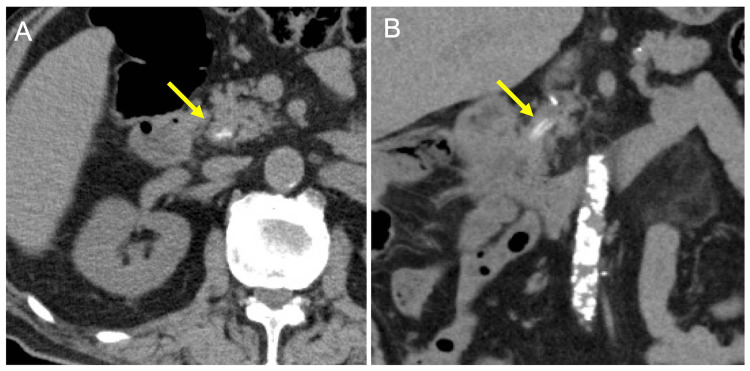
CT scan upon admission for obstructive jaundice. A CT scan at the level of the papilla of Vater reveals a high-density lesion in the distal bile duct (arrow) on the axial (A) and coronal (B) images. CT, computed tomography
